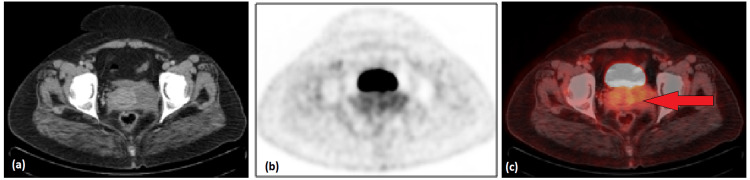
Interim pelvic CT (a), PET (b) and PET CT fusion (c) axial images showing good partial treatment response with interval regression in size and metabolic activity of the cervix/vaginal mass (red arrow).
